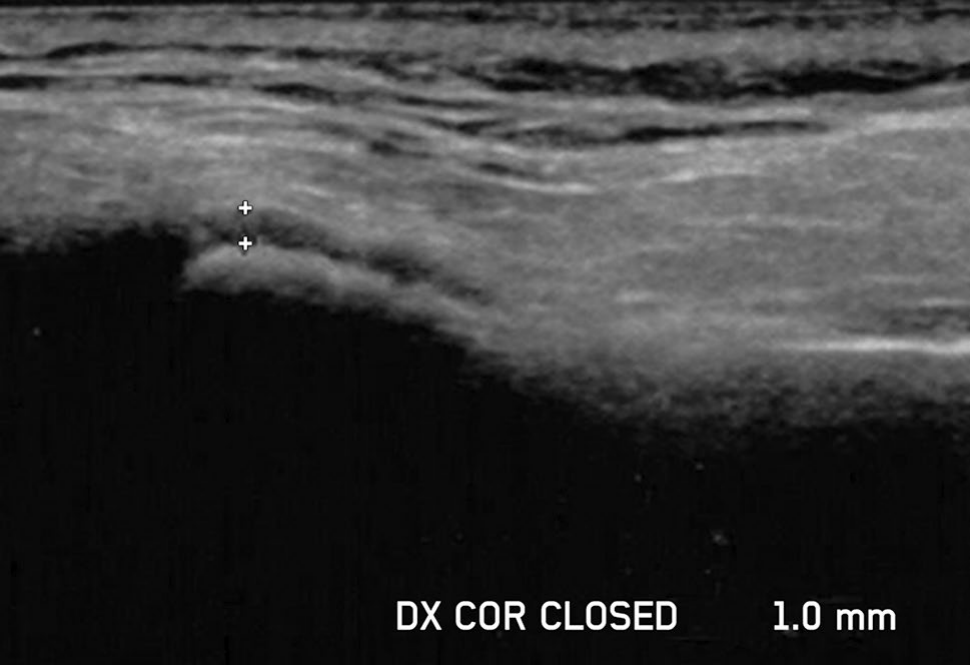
Coronal ultrasound image of the right temporomandibular joint obtained in closed-mouth position. The two reference point markers identified the hypoechoic stripe above the most lateral point of the condylar cortex, corresponding to the width of the lateral periarticular space
